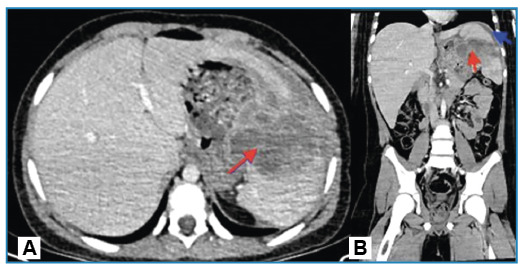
Axial- (A) and coronal-plane (B) CT images of a hematoma occurring in the perisplenic region one month after the drainage. 7x5-cm septated and wall-enhancing collection area, extending into the splenogastric recess (red arrows); free fluid measuring 15 mm in depth in the perisplenic space (blue arrow).
